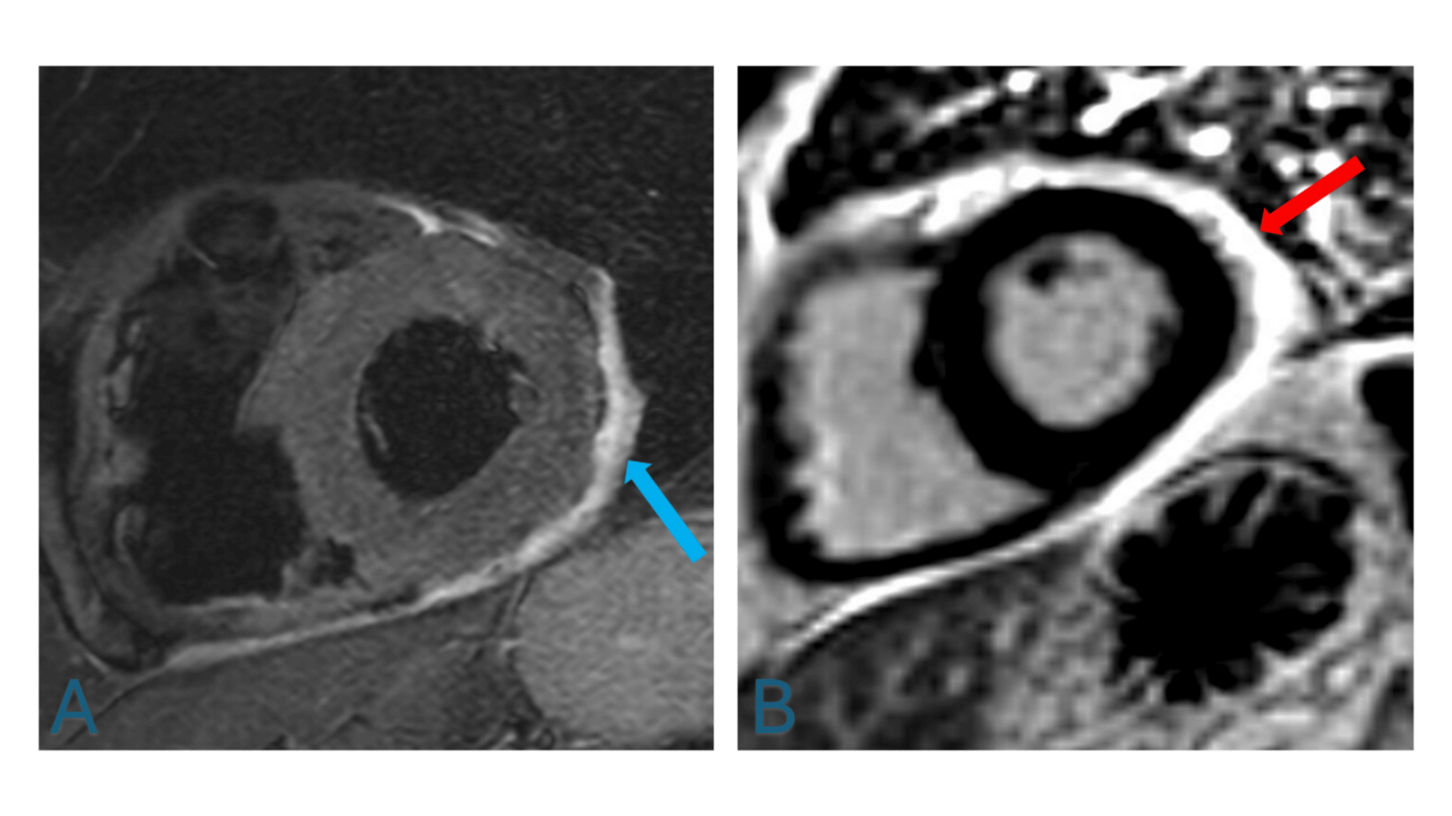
Cardiac Magnetic Resonance Imaging Short axis short tau inversion recovery (STIR) fluid-sensitive (A) and delayed post-gadolinium contrast cardiac MRI sequences (B). Note the bright, hyperintense signal on the STIR sequence indicating pericardial edema (blue arrow) and late post-gadolinium contrast enhancement involving the thickened pericardium (red arrow), consistent with pericarditis and associated pericardial effusion.
